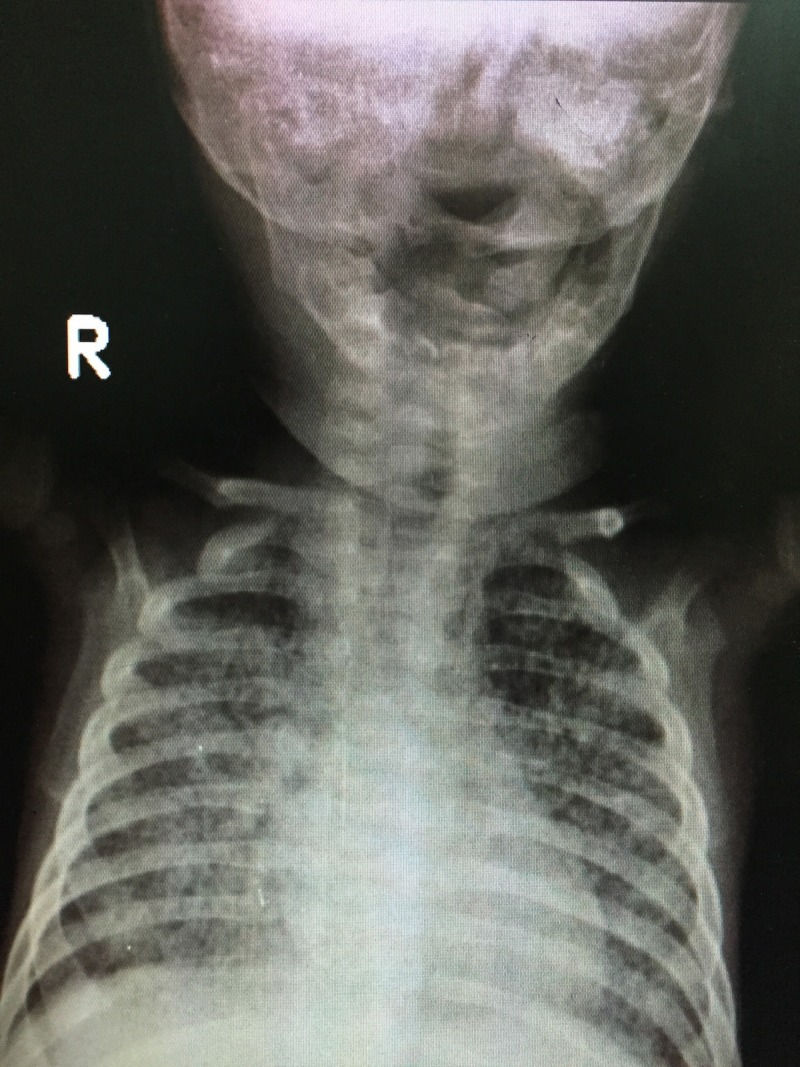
Anteroposterior (AP) view of skull and mandible Radiograph shows no cortical thickening or periosteal new bone formation in the mandible. Bilateral clavicles appear normal.
